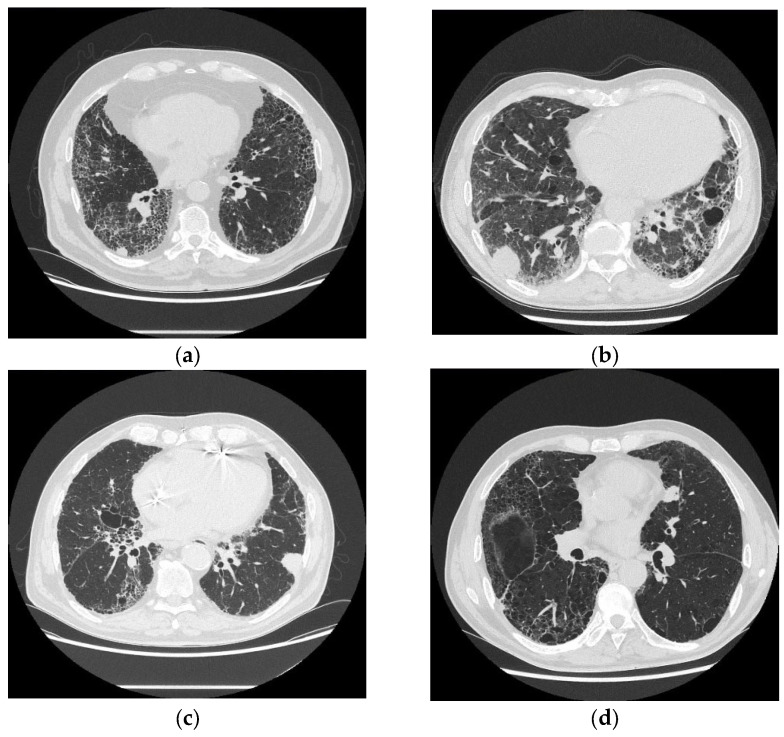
Examples of LC in patients with IPF on HRCT imaging. Panels (a–c): HRCT of the IPF-LC patients. Panel (d): HRCT of the CPFE-LC patient.
